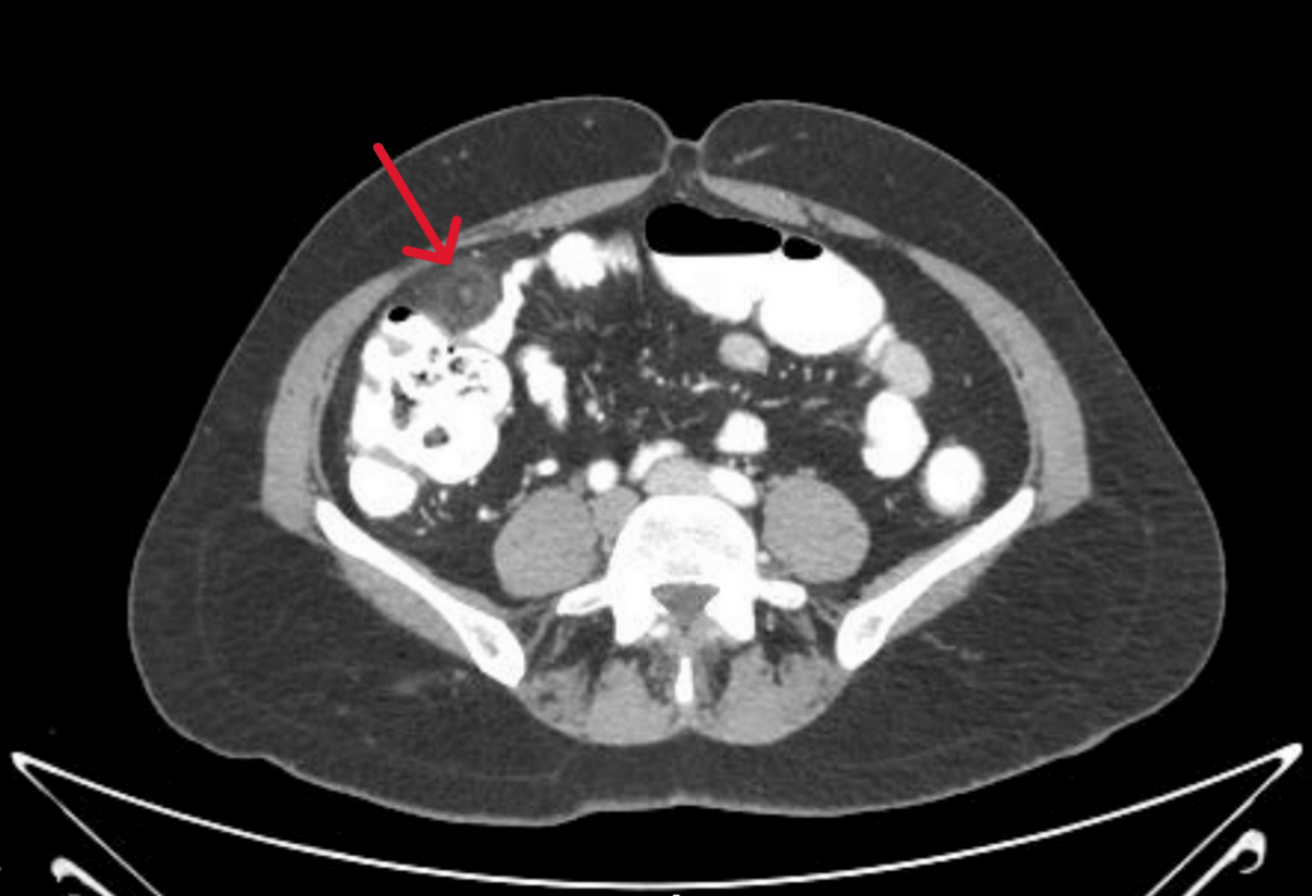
Contrast-Enhanced CT Revealing Epiploic Appendagitis Mimicking Acute Appendicitis (Case 4) Axial CECT image demonstrates a well-defined, oval, fat-attenuating lesion with a thin hyperattenuating rim (red arrow) adjacent to the ascending colon. The appendix appears normal in a retrocecal location. These findings are characteristic of epiploic appendagitis* *— a benign mimic of acute appendicitis.
